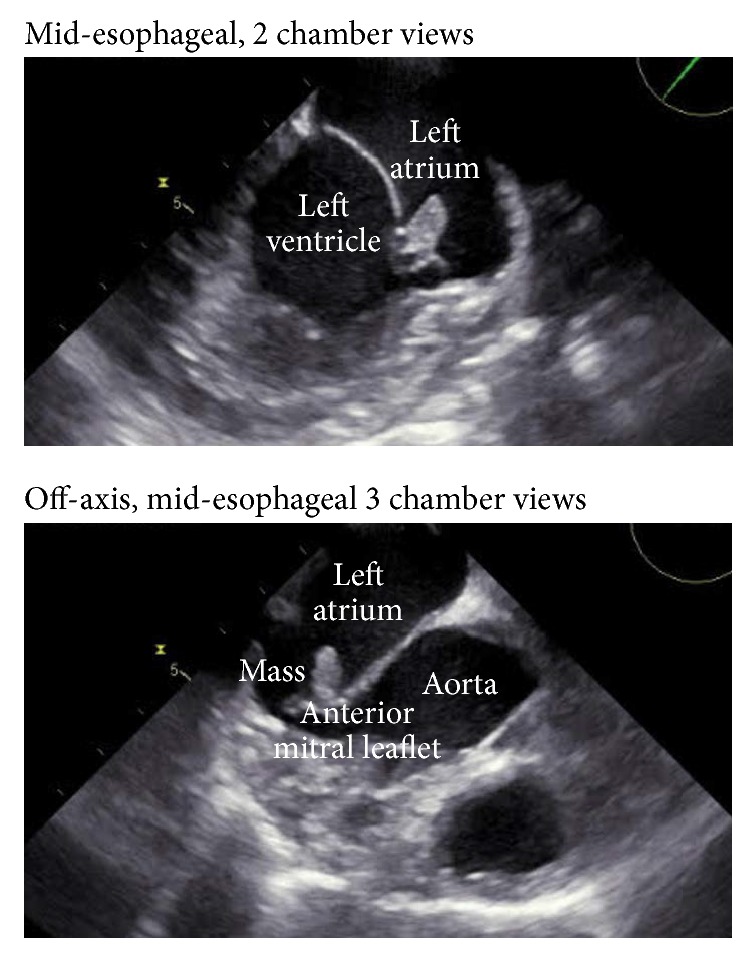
Transesophageal echocardiogram (TEE) image showing a pedunculated mass on the atrial aspect of the posterior leaflet of the mitral valve before surgical resection of the CPFE.
